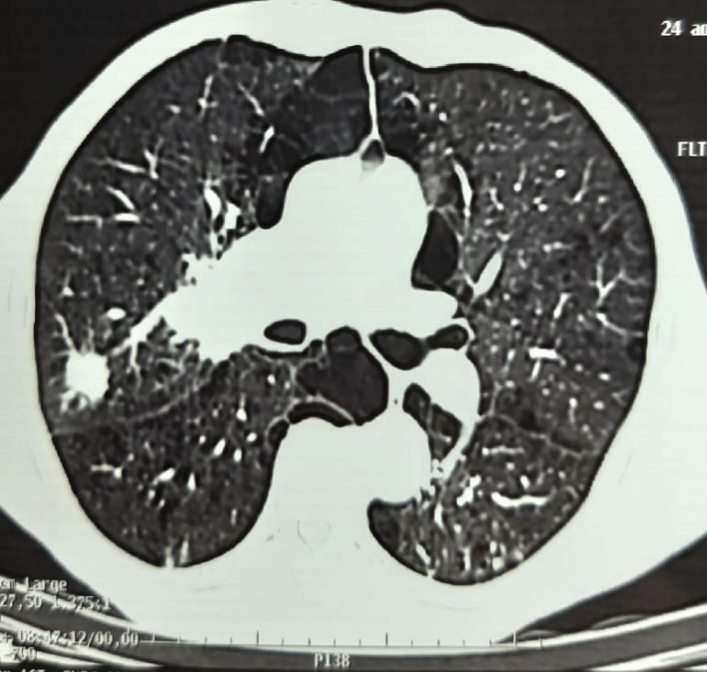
The thoracic computed tomography (CT) showed a right hilar tumoral process extended to the posterior segment of the right upper lobe of irregular and spiculated contours, associated with a spiculated nodule of the right upper lobe.
